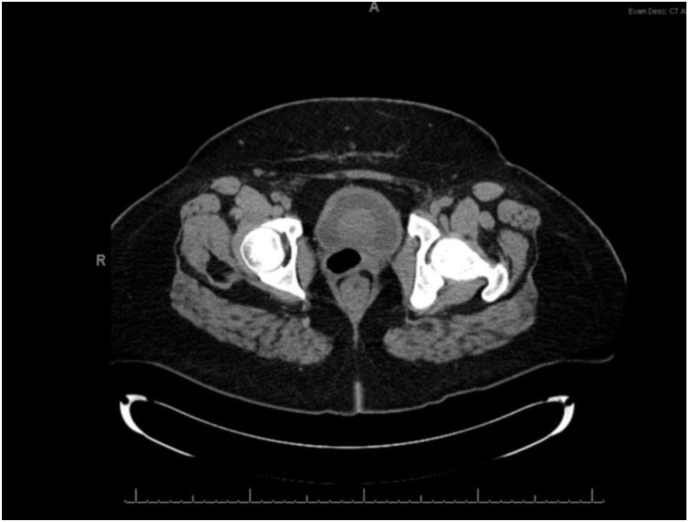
Contrast-enhanced magnetic resonance imaging T2 weighted signal revealing an ovoid smooth mass at the bladder base and neck with hypointense T2 signals, with no transmural extension.
